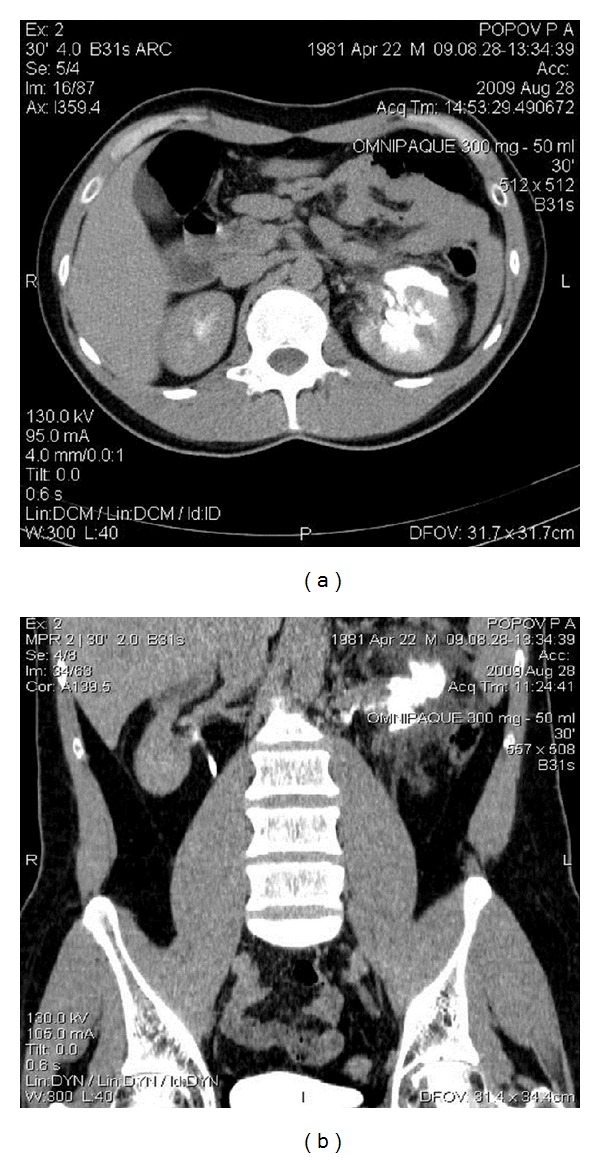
Computed tomography showing extravasation of the contrast medium through the gap in pelvis—spontaneous rupture. Axial (a) and sagittal (b) images.
